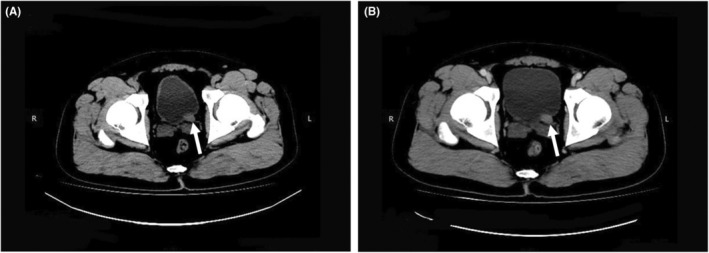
Computed tomography of small cell neuroendocrine carcinoma of the ureter. (A) Plain computed tomography scan showed a soft tissue mass of the lower ureter that was heterogeneous and well‐defined, which grew into the bladder cavity (arrow). (B) Enhanced computed tomography showed the soft tissue mass of the lower ureter that was slightly inhomogeneous enhancement (arrow).
